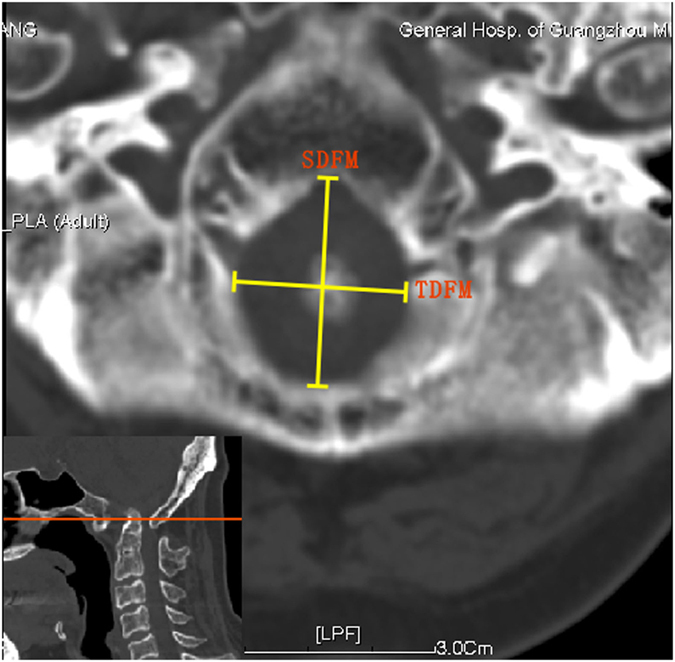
Diameter measurements of foramen magnum on CT images. SDFM, sagittal diameter of foramen magnum; TDFM, transverse diameter of foramen magnum.
